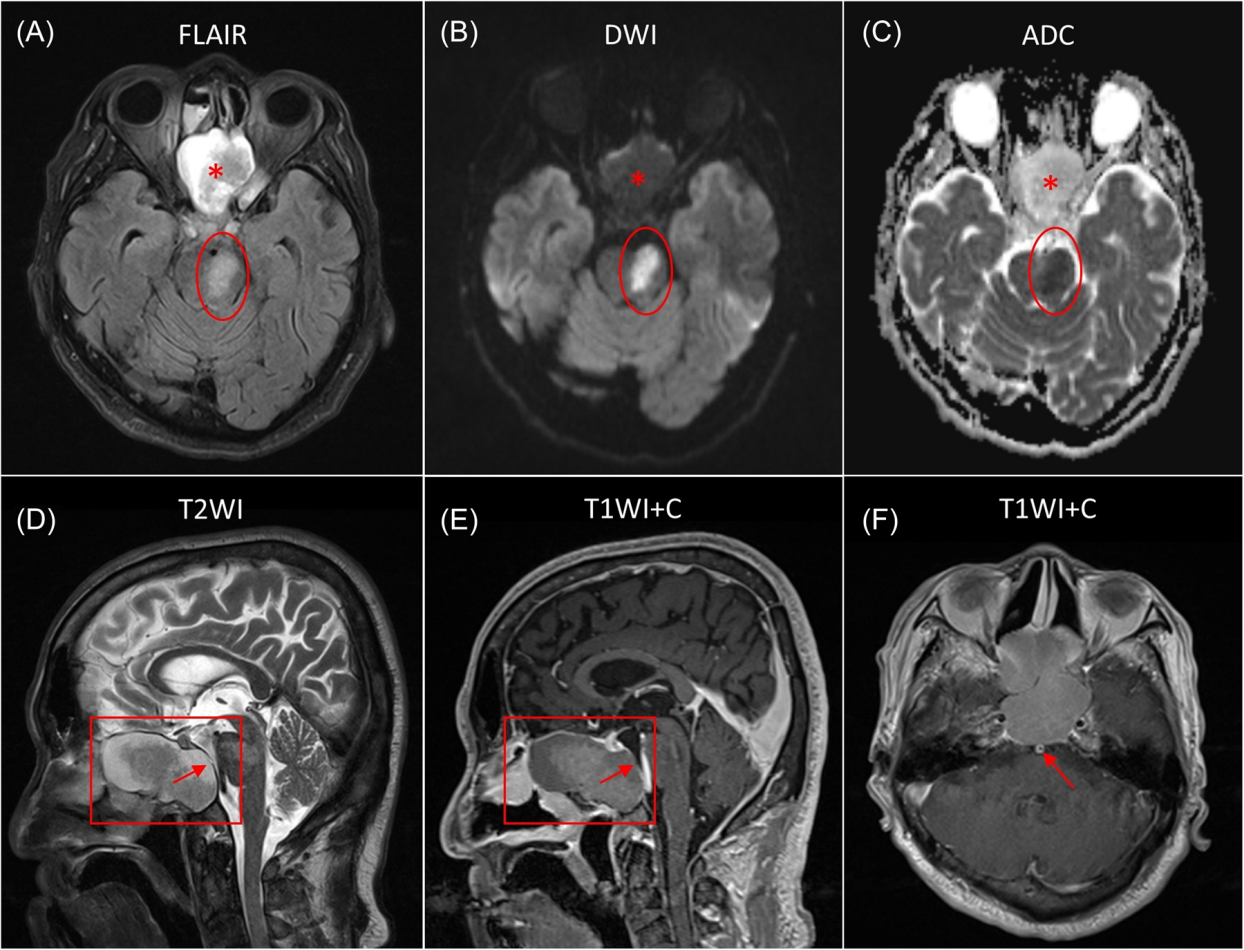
MRI: (A) Left pontine infarct and sphenoid mucocele (hyperintensity); (B) DWI shows restricted diffusion; (C) ADC map shows facilitated diffusion; (D, E) Sagittal images reveal expansile lesion and mucosal thinning; (F) Wall enhancement of basilar artery.
